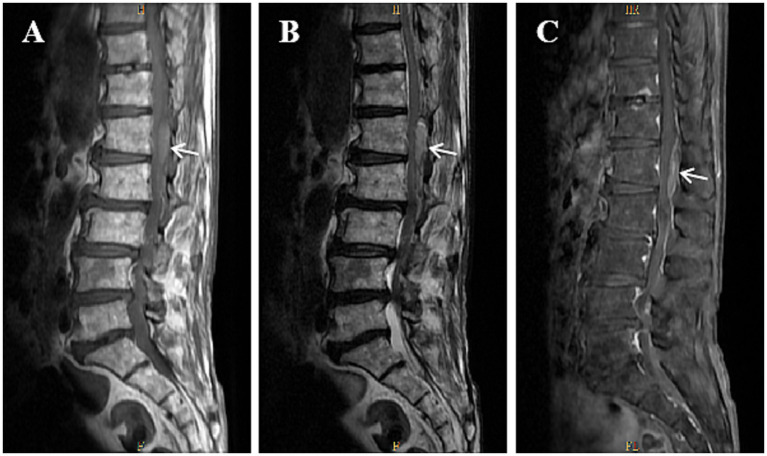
Magnetic resonance imaging (MRI) of the lumbar spine at the L1 level. (A) Sagittal T1-weighted image shows a 29 × 9 mm spindle-shaped, slightly hyperintense lesion within the spinal canal (white arrow). No abnormal paraspinal soft tissue signal is noted. (B) Sagittal T2-weighted image demonstrates the lesion with similar morphology and slightly hyperintense signal (white arrow). (C) Contrast-enhanced T1-weighted image reveals no significant enhancement of the lesion, suggestive of a hematoma (white arrow).
